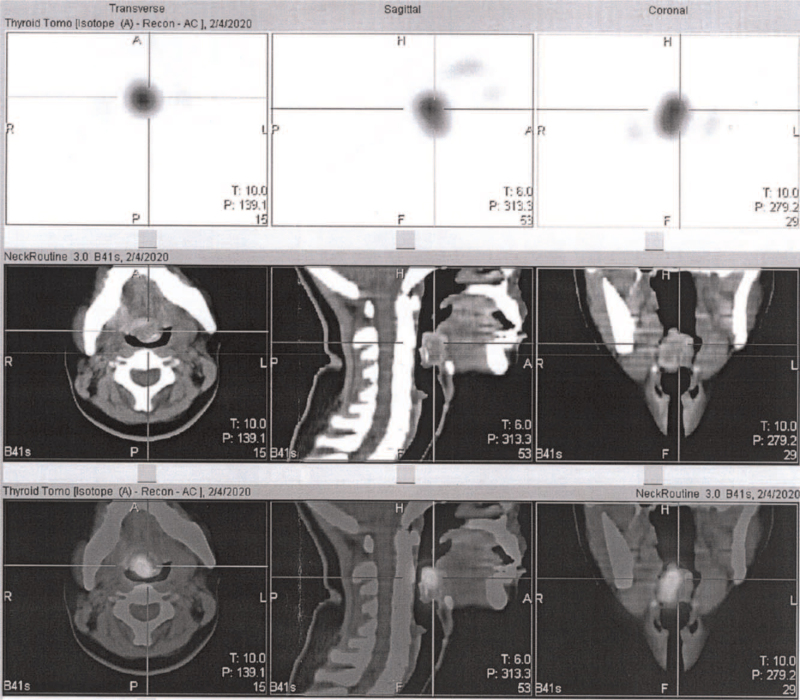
Thyroid scintigraphy with technetium (^99m^Tc) showed uptake of the radiotracer at the base of the tongue. Lingual thyroid was detected. No uptake was seen in the normal thyroid gland location.
